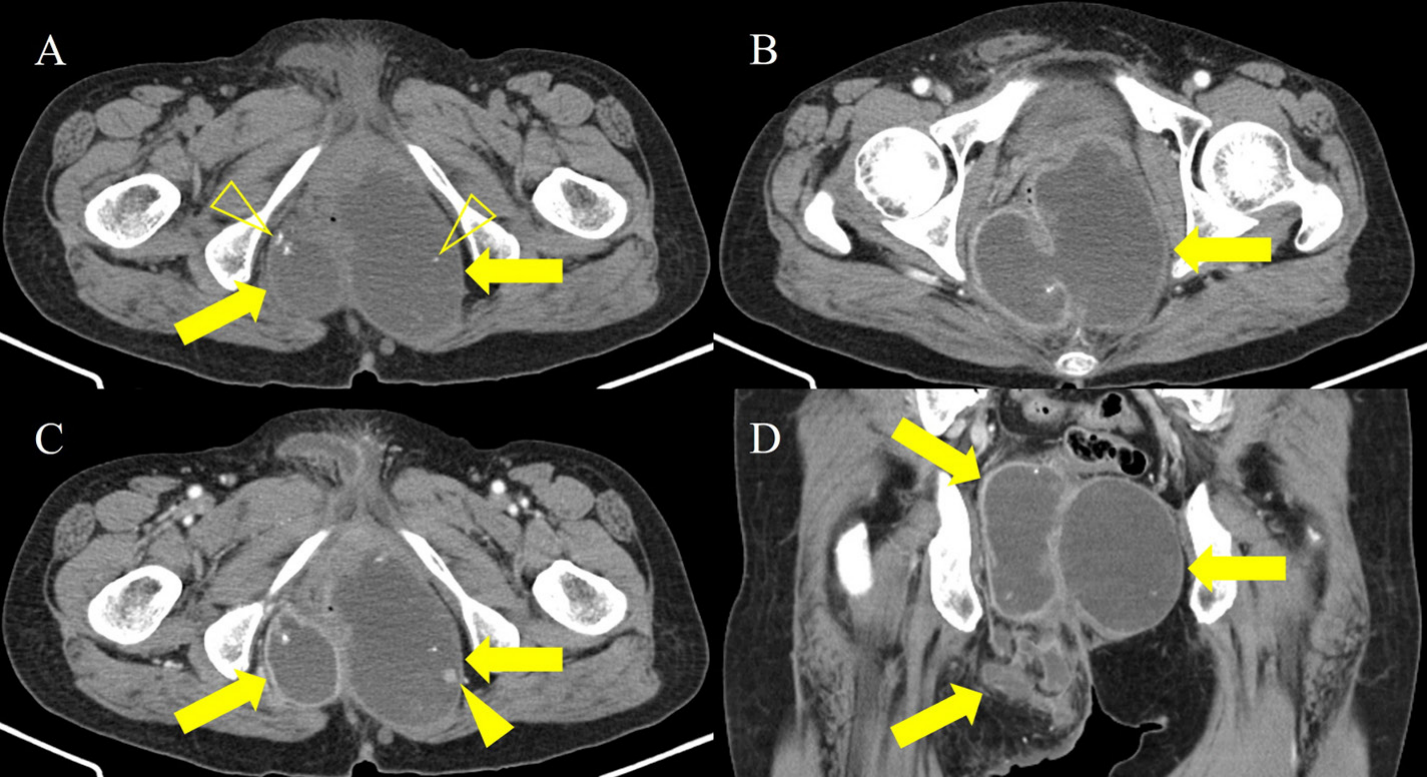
CT images demonstrating multilocular cystic mass around the rectum and anus.
